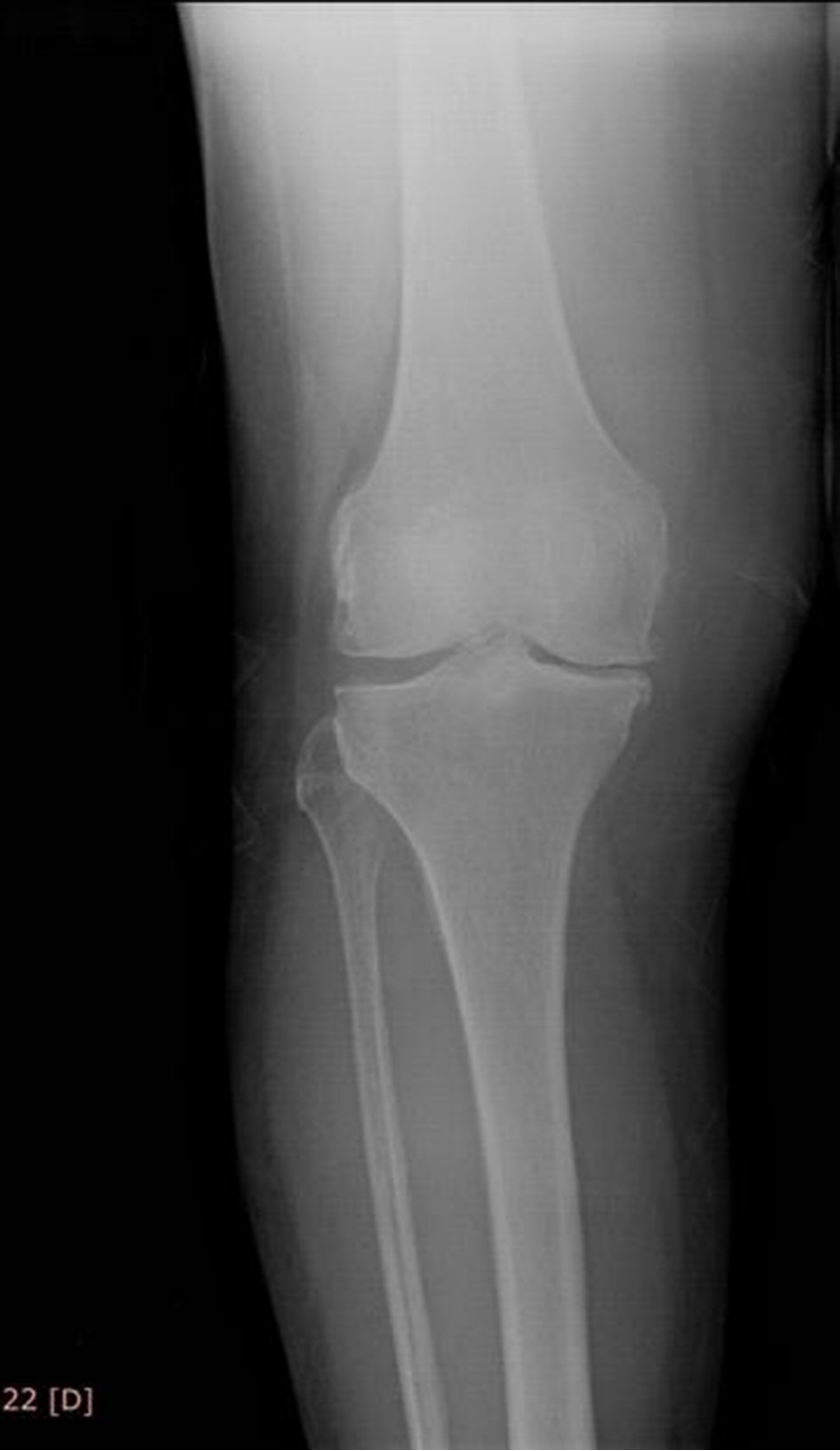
Knee Radiography After Prolotherapy
